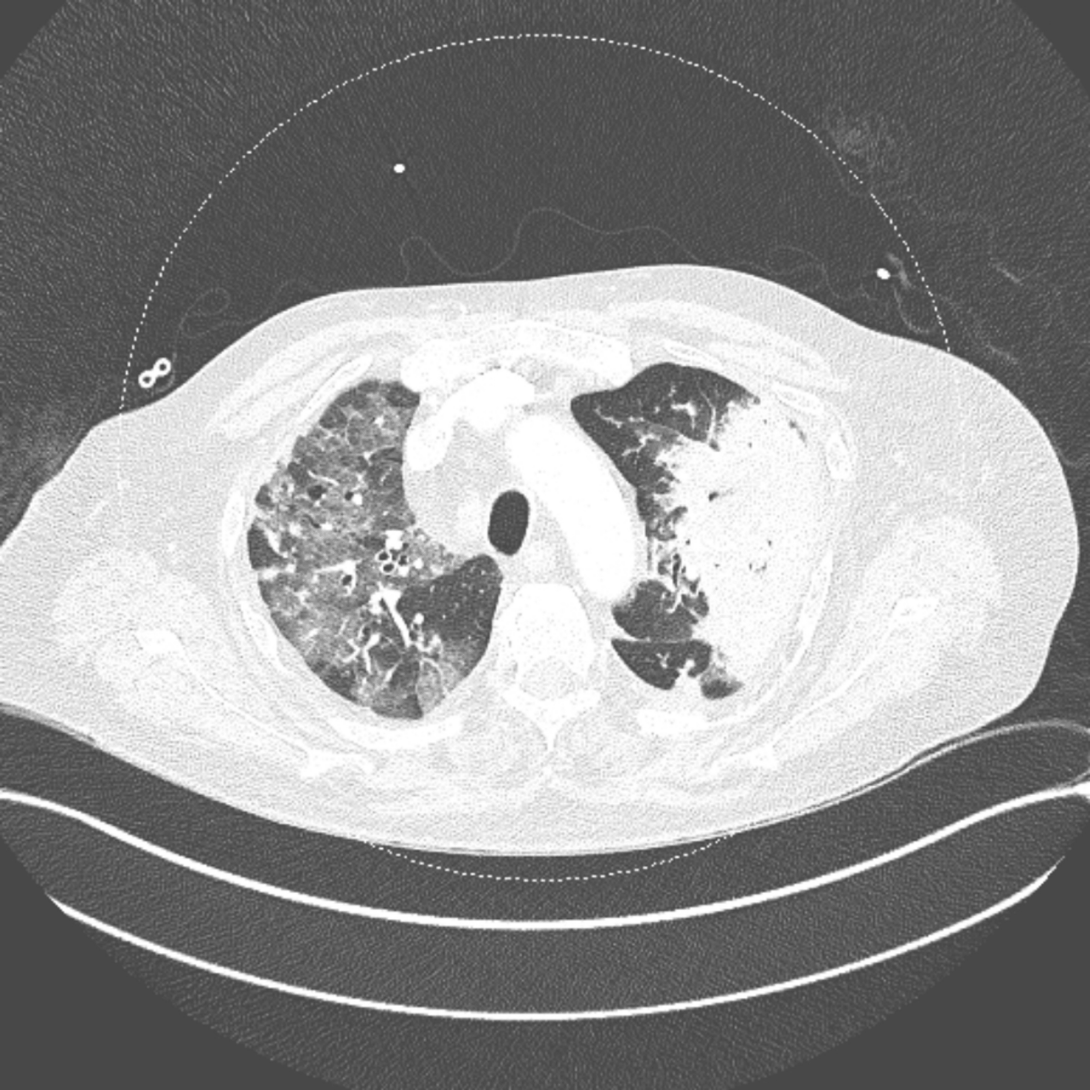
CT pulmonary angiogram two weeks after the admission showing worsening picture CT: computed tomography
